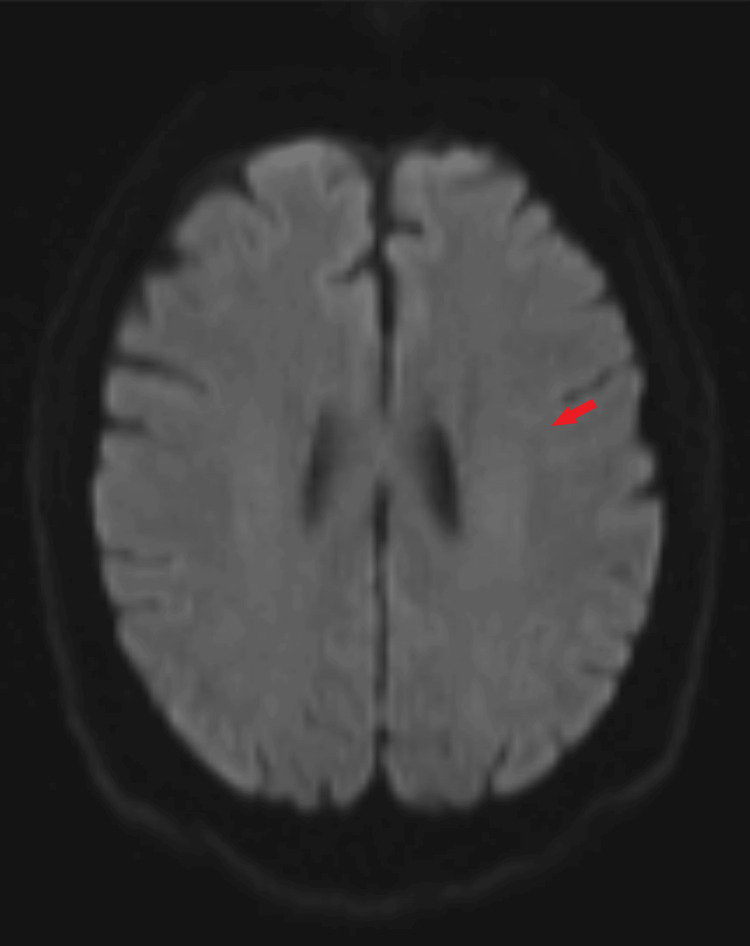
Diffusion-weighted imaging (DWI) axial brain magnetic resonance imaging (MRI) shows no evidence of acute intracranial abnormality in the posterior aspect of the left frontal lobe as the region of interest. This region of interest is marked with a red arrow.
